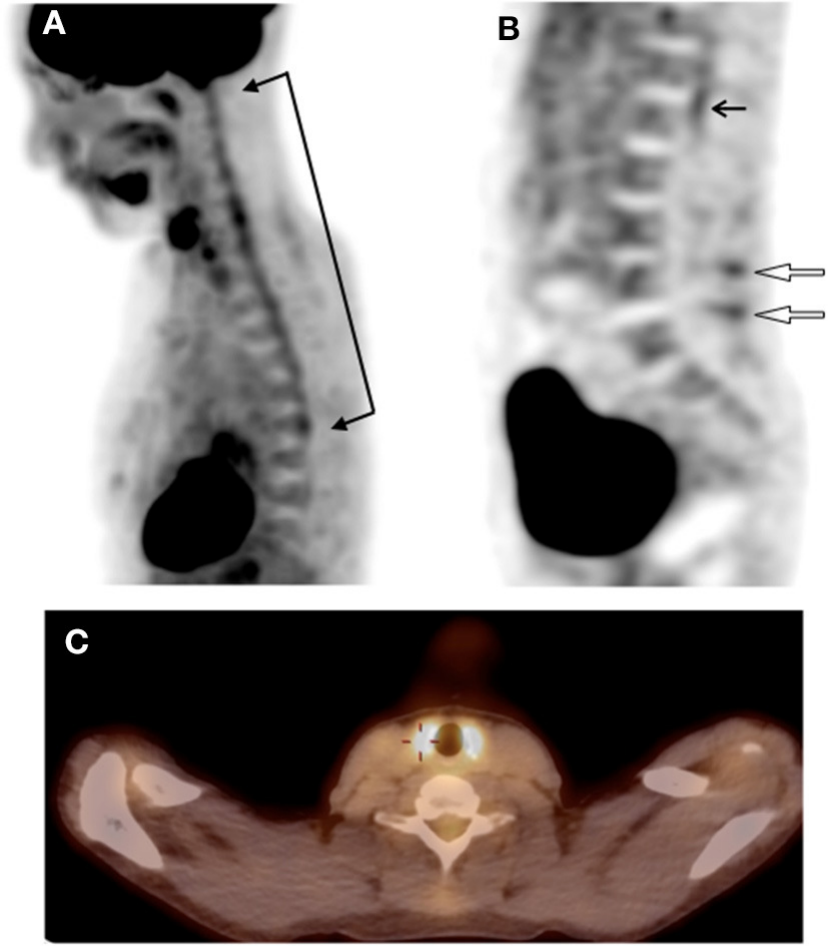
(A) sagittal view of FDG-PET/CT showing hypermetabolism of the spinal cord at the cervicothoracic level (between arrows). (B) sagittal view of FDG-PET/CT showing hypermetabolism of the spinal cord at the lumbosacral level (black arrow) and an interspinous hypermetabolism at two level of the lumbar spine (open arrows). (C) fused FDG-PET/CT image on the transverse plane showing a marked and diffuse hypermetabolism of the thyroid gland. FDG-PET/CT, whole body 19-fluorodeoxyglucose positron emission tomography with computed tomography.
